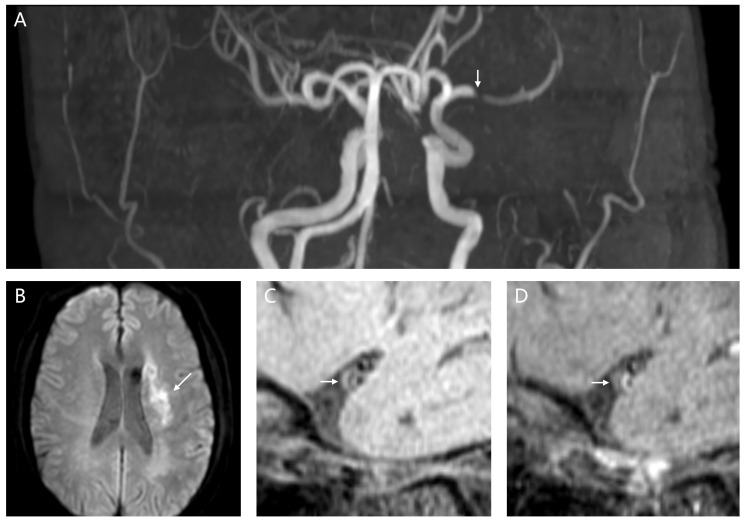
Identification of symptomatic MCA atherosclerotic plaque. (A) A symptomatic stenosis of the left MCA-M1 segment on magnetic resonance angiography imaging (white arrow). (B) Acute infarct in the left MCA-M1 territory on diffusion-weighted imaging (white arrow). (C,D) An eccentric, focal plaque in the left MCA-M1 segment as a symptomatic atherosclerotic lesion before and after administrating contrast on T1-weighted imaging, respectively (white arrows). MCA, middle cerebral artery (scale bar = 10 mm for (A,B); scale bar = 5 mm for (C,D)).
